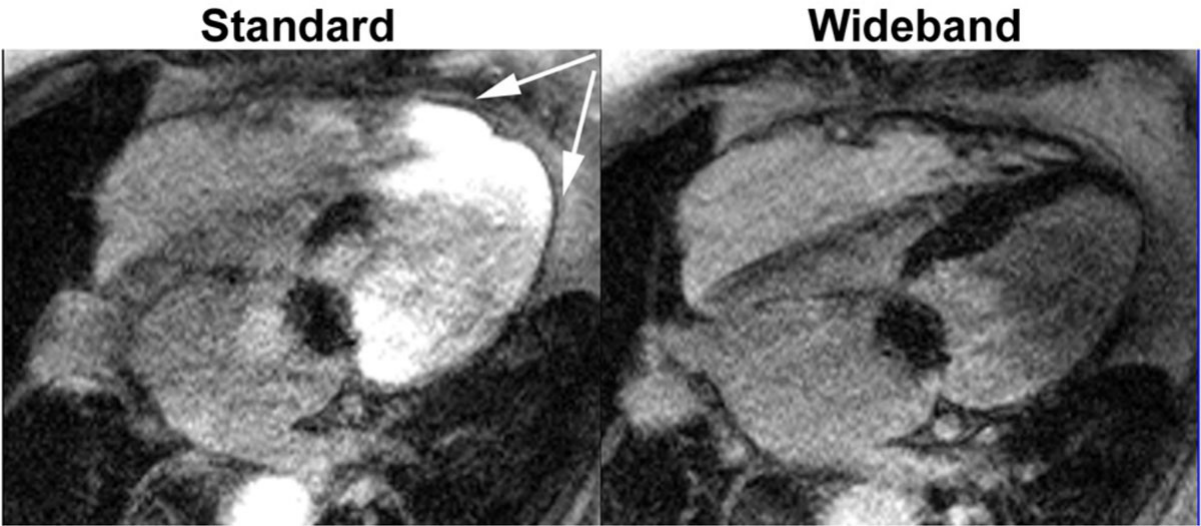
(Left) Standard and (right) wideband LGE images of a patient with ICD acquired at 1.5T. Compared with standard LGE MRI which exhibited significant artifacts (arrows), wideband LGE MRI suppressed image artifacts induced by an ICD and enables visualization of hyper-enhancement in the thinned lateral wall with higher diagnostic confidence.
